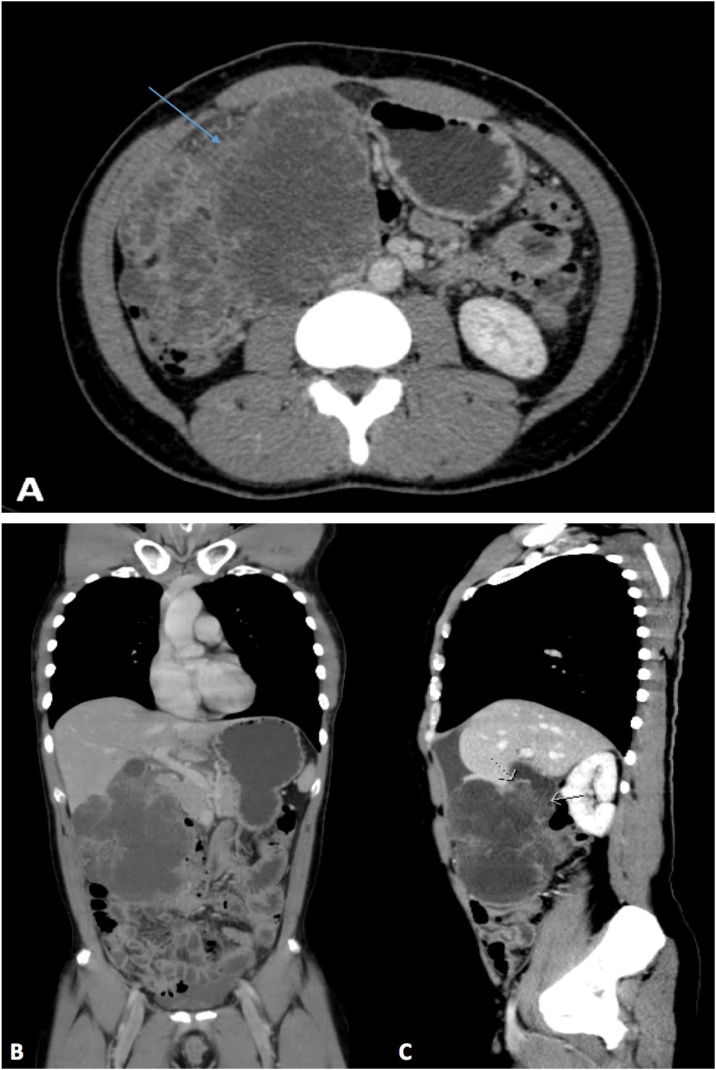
A: Computed tomography scans of the chest and the abdomen revealing a large polypoidal enhancing mass in the gallbladder measuring 11.5 × 9.2 × 5 cm with focal lesions in segment V. B: Coronal view of computed tomography scans of the chest and abdomen revealing a large gallbladder mass. C: Sagittal view of the same image.
